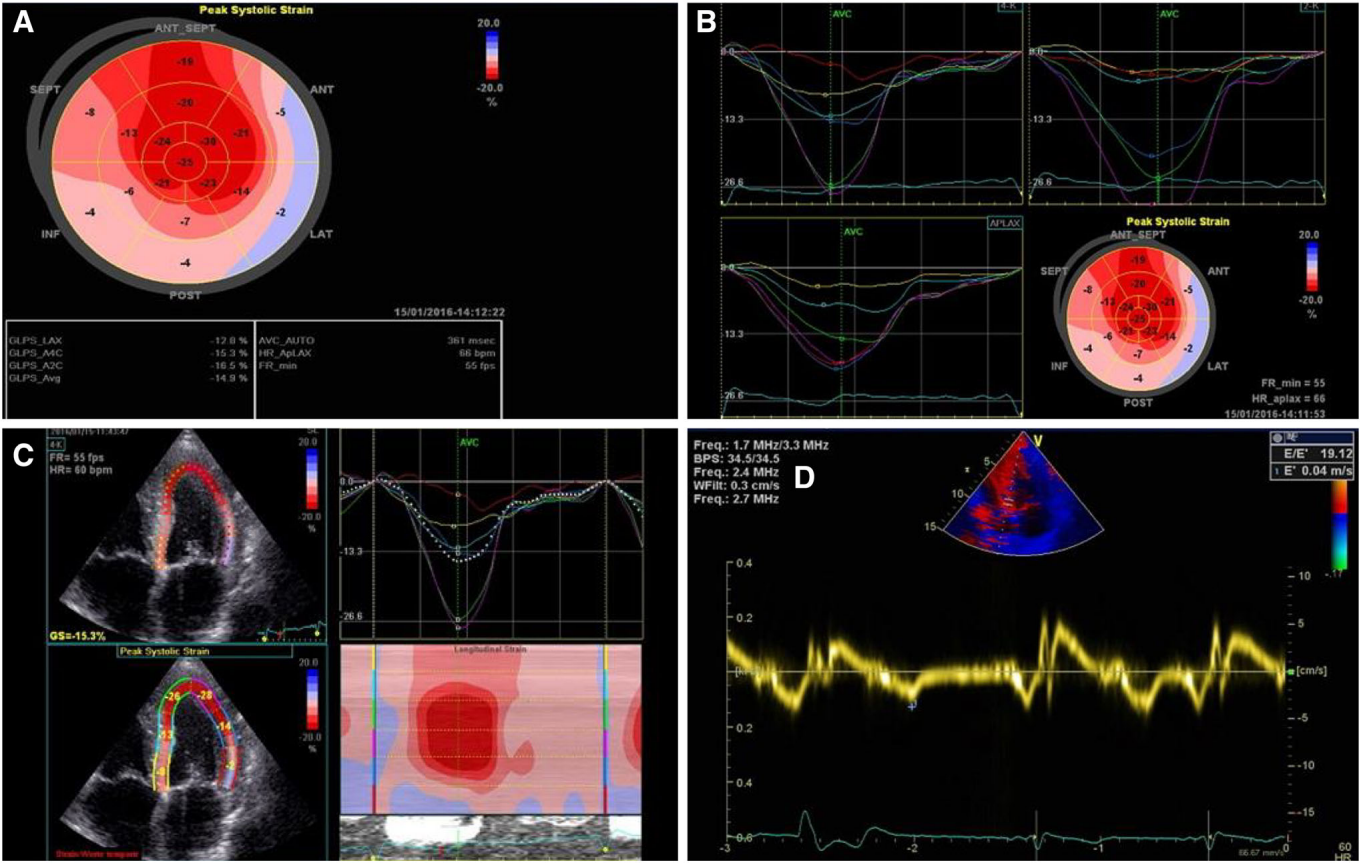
a–c 2D strain analysis with apical sparing as a typical sign for amyloidosis. d Spectral tissue Doppler (TDI) shows an antegrade systolic and two retrograde waves (*E*′ and *A*′). *E*/*e*′ measured 19.12 as an indication for a severe diastolic dysfunction
